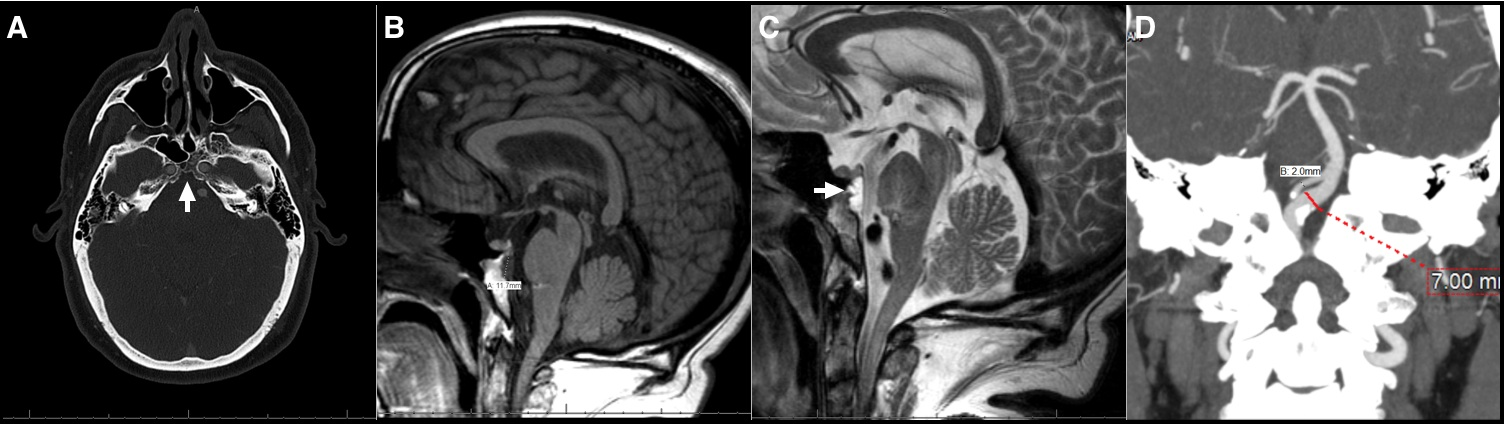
Brain Radiography for Patient 2 (A) Axial CTA (bone window); (B) Sagittal T1 MRI; (C) Sagittal T2 MRI showing EP and pontine telangiectasia; (D) CTA showing fenestrated basilar artery
